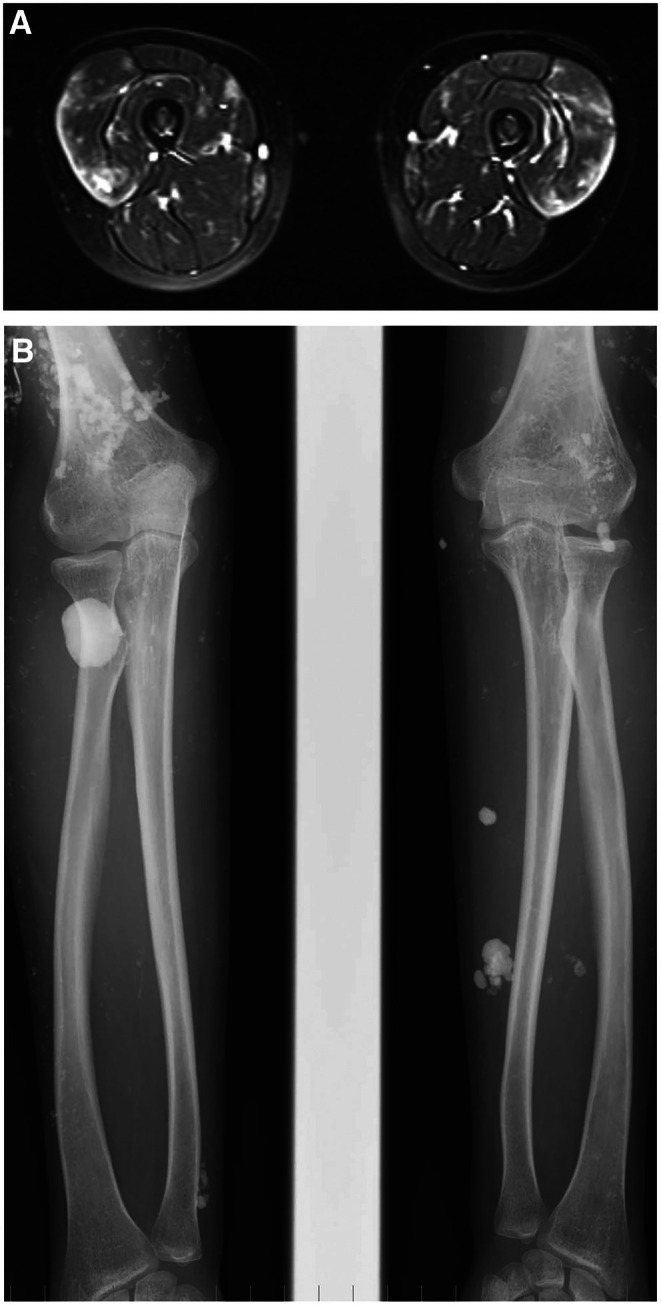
(A) A 6‐year‐old girl with juvenile dermatomyositis presenting with erythema and Gottron's papules without overt clinical symptoms of myositis. Fat‐suppressed T2‐weighted MRI showed bilateral hyperintensities in the thigh extensor muscles, suggestive of active myositis. (B) An adult male with multiple calcinosis lesions, who developed juvenile dermatomyositis at age 6. Multiple calcinosis lesions are present in the subcutaneous tissues of both forearms. The images were kindly provided by Dr. Taiki Nozaki, National Defense Medical College.
